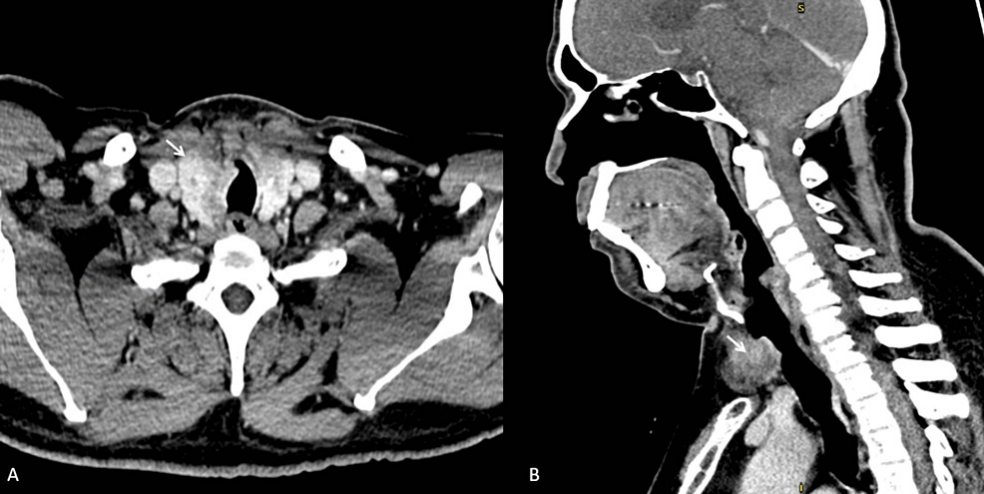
Computed tomography of the neck showing thyroid lesion with tracheal involvement A: Axial cut with arrow showing tumor with tracheal cartilage invasion B: Sagittal cut with arrow showing tumor
